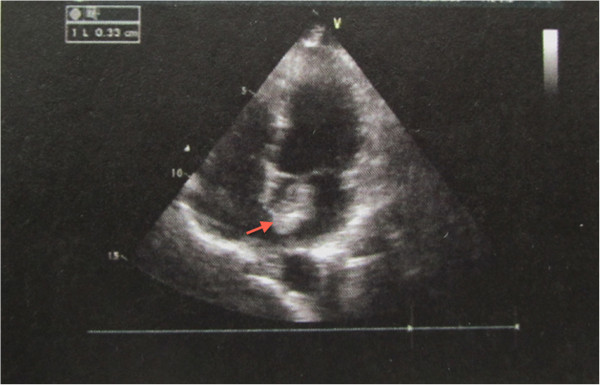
Echocardiography showing atrial mass during systole (red arrow): septal left atrial side with a hyperechoic mass (1.9 × 2.8 cm), pedunculated in the atrial septum with a pedicle length of 0.3 cm.
